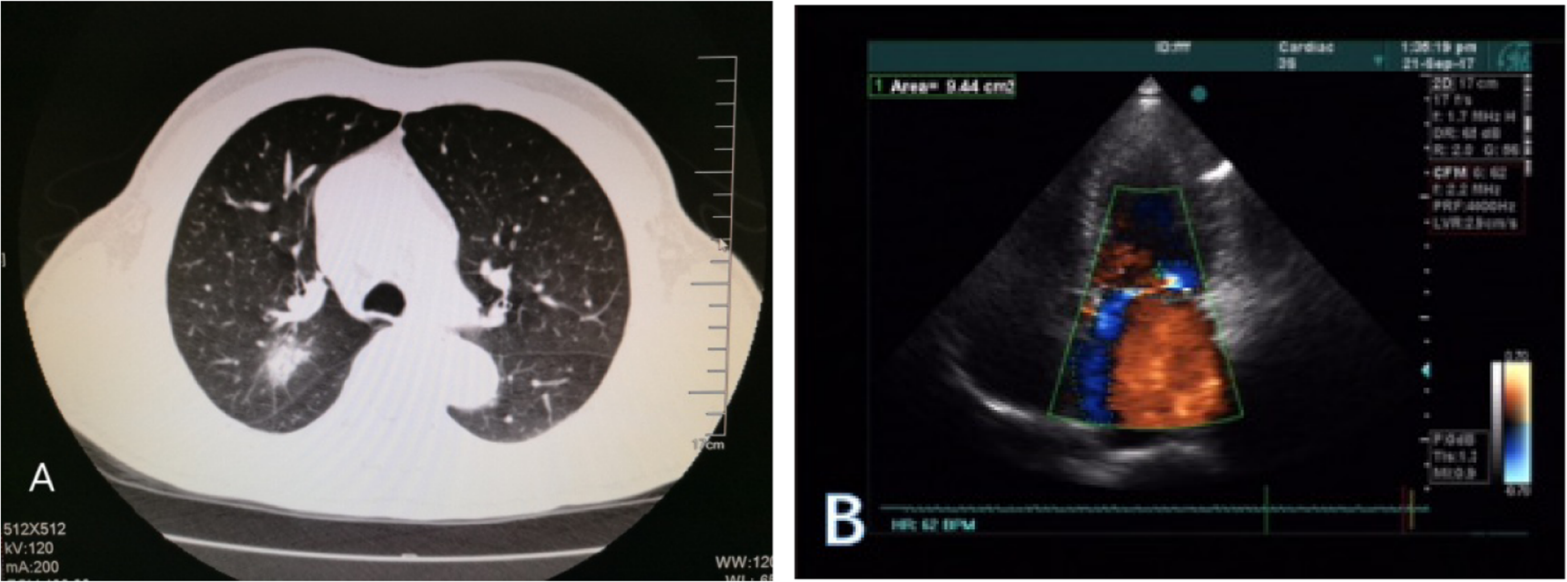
a Computed tomography imaging scans identified a right upper lobe apical mass. b Transthoracic echocardiographic imaging of Mitral prolapse associated with severe MR
